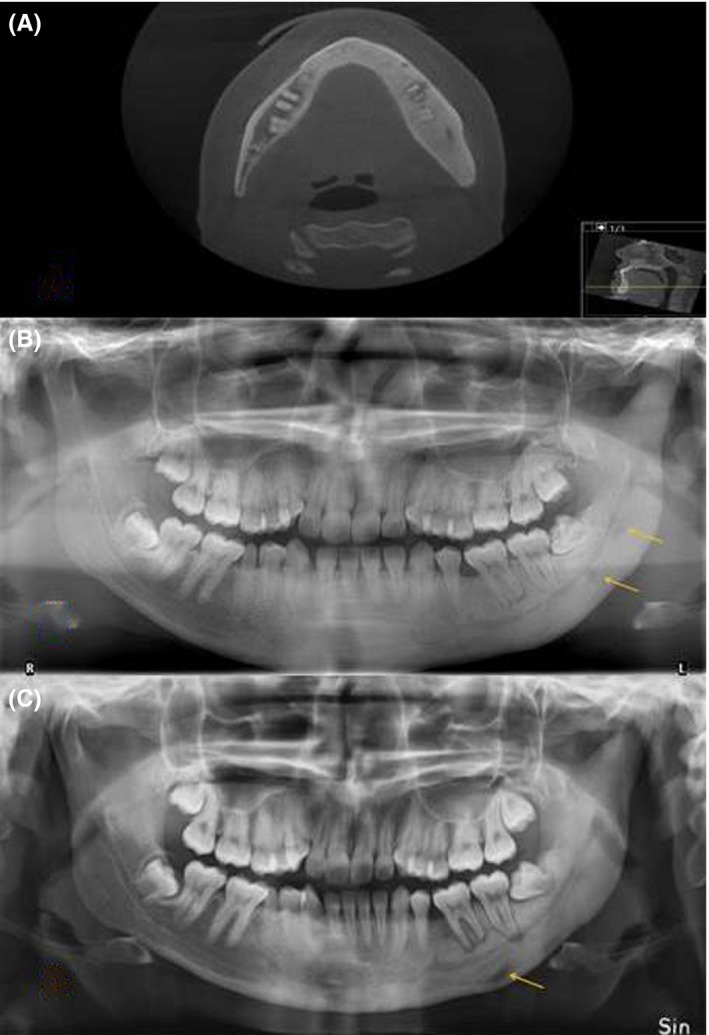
Radiological examinations of a 21‐year‐old woman with diffuse sclerosing osteomyelitis of the left side of the mandible treated with cortisone and analgesics for five years. A, CT before the treatment with denosumab, revealing sclerosis of the entire left side of the mandible, crossing the midline, and some thickening of the mandible and widening of lamina dura around teeth 36, 37, and 38 as typical signs of diffuse sclerosing osteomyelitis. B, Orthopantomogram before the treatment with denosumab, showing sclerosis of the left side of the mandible with areas of radiopaque areas, and some bone apposition. C, Orthopantomogram after 20 mo with denosumab treatment showing more radiolucency around teeth 36, 37, and 38, indicating bone resorption but somewhat less sclerosis. The teeth 36, 37, and 38 had been vital throughout the treatment period
